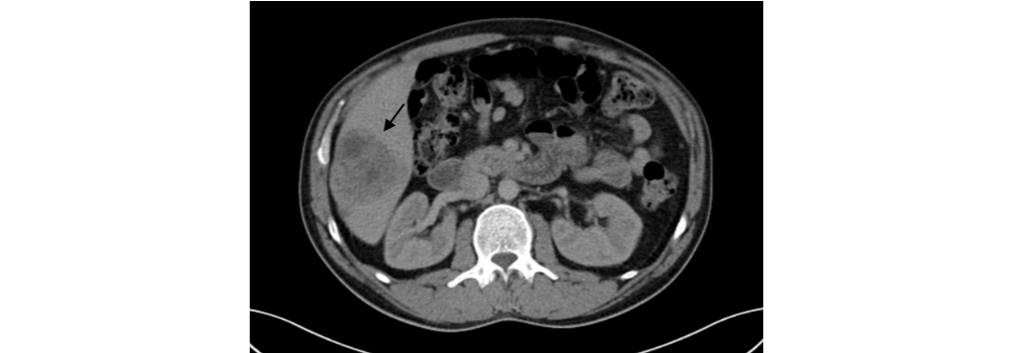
Thoraco-abdomino-pelvic CT scan showing hepatic lesions. CT: computed tomography.
